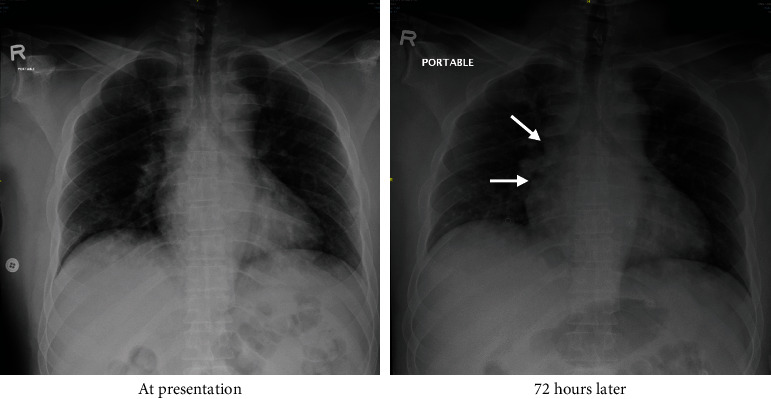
Chest radiograph on the day of onset of symptoms showing no evidence of pneumonia nor any radiological findings of acute respiratory distress syndrome (ARDS). At 72 hours: ground-glass opacification and alveolar consolidation were seen in the middle of right lobe (arrows).
